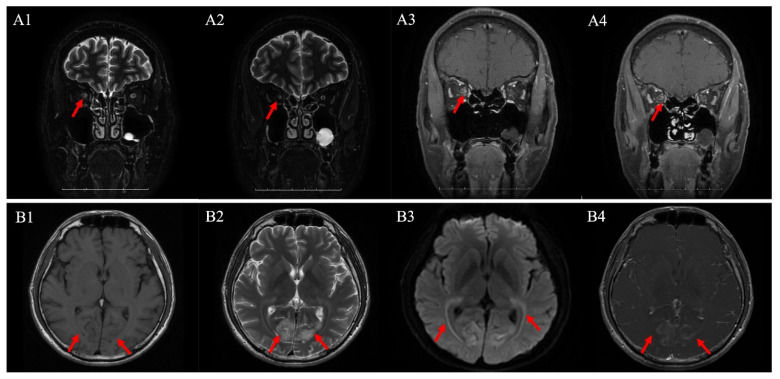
Orbital and cranial MRI findings of Case 11. A, Orbital MRI: Coronal short tau inversion recovery (STIR) sequences (A1,A2) and gadolinium-enhanced T1-weighted imaging (T1WI) (A3,A4) demonstrate hyperintensity in the intraorbital, intracanalicular, and intracranial segments of the right optic nerve (red arrows); the left optic nerve shows normal morphology and signal intensity. B, Cranial MRI: Patchy lesions are visualized in the bilateral occipital cortex and subcortical white matter, manifesting as hypointensity on T1WI ((B1), red arrow), hyperintensity on T2WI ((B2), red arrow), restricted diffusion on diffusion-weighted imaging (DWI) ((B3), red arrow), and significant enhancement on gadolinium-enhanced T1WI ((B4), red arrow).
